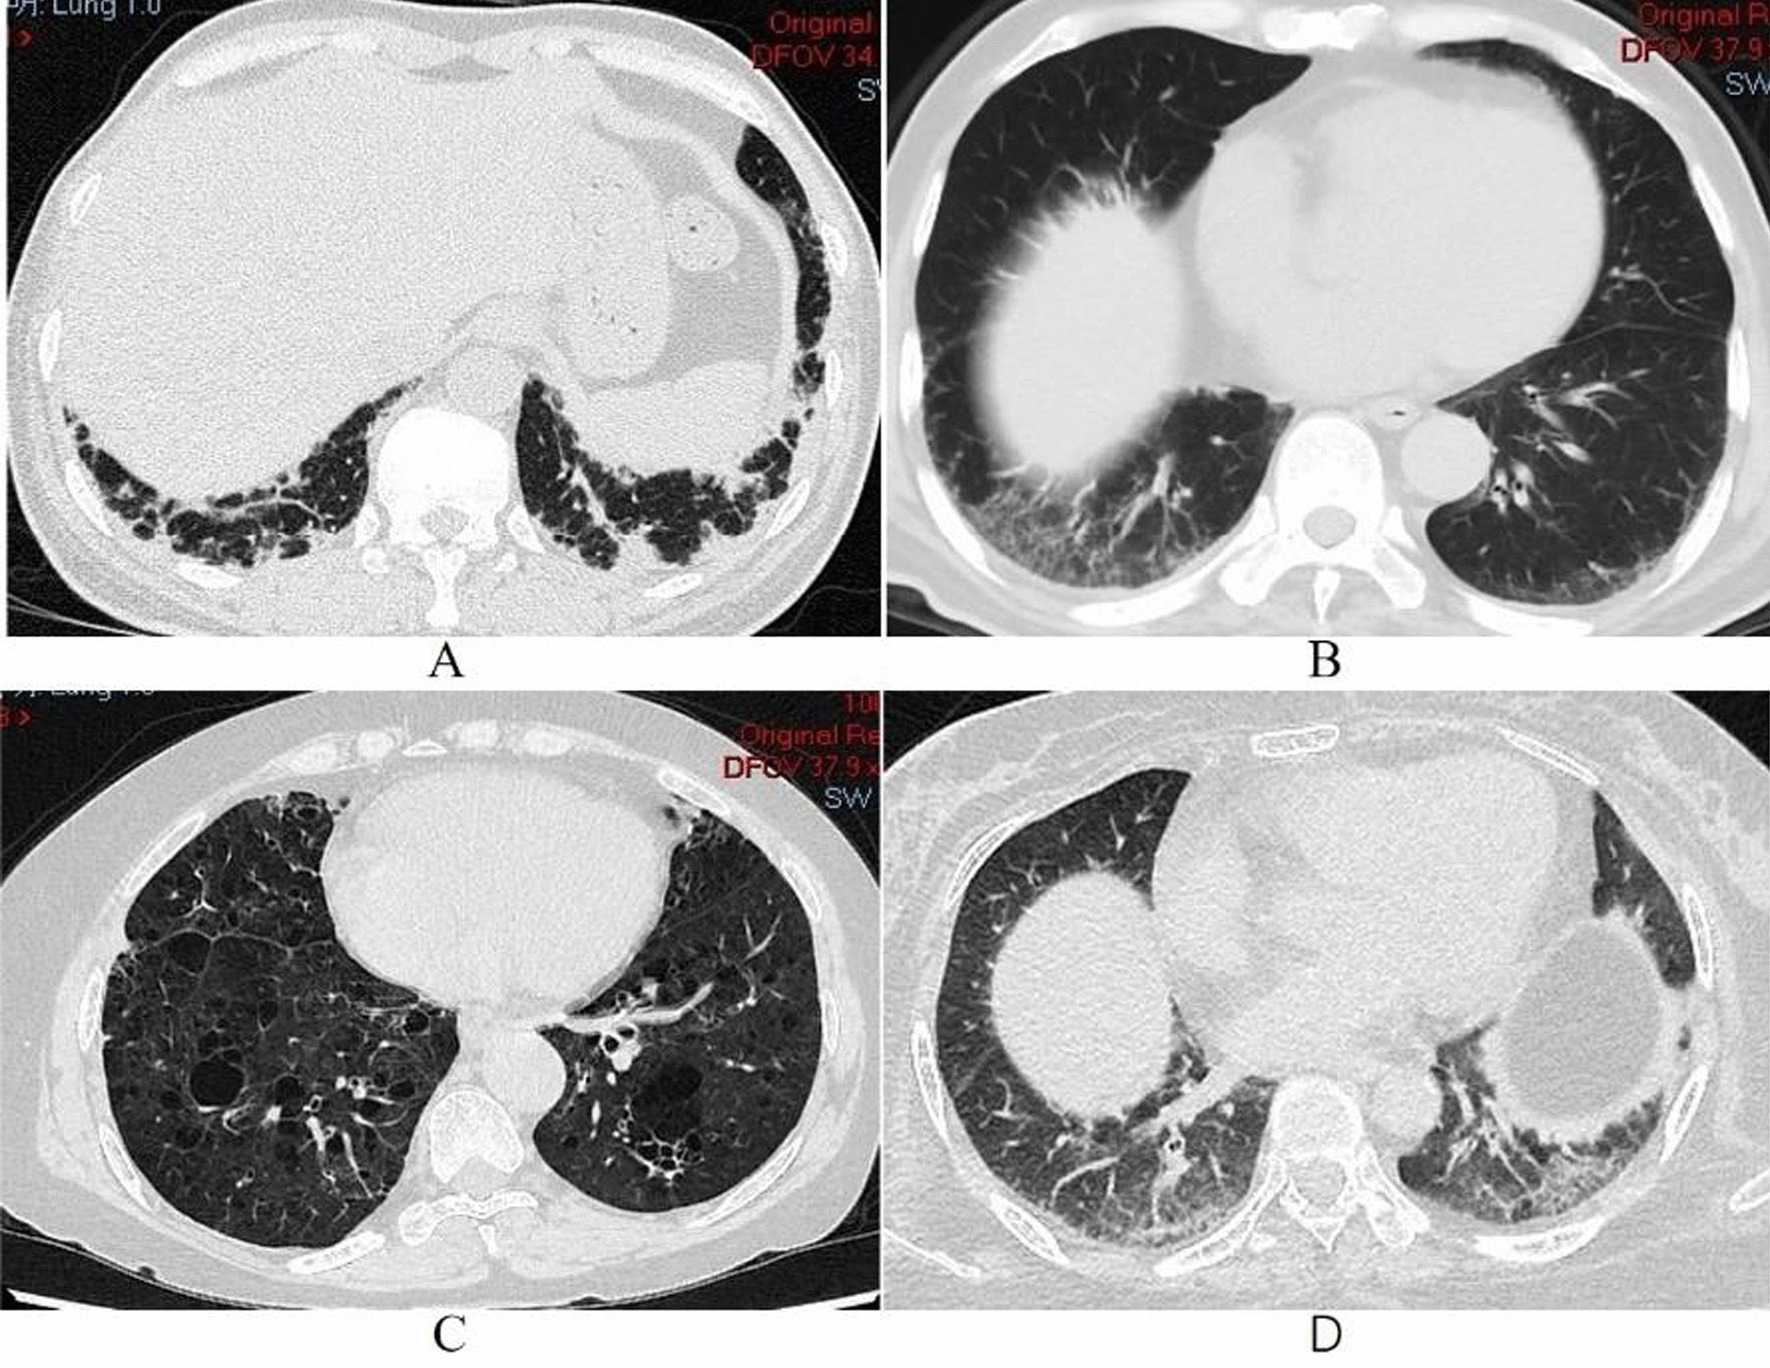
Abnormal opacities of HRCT from different SRP-IMNM patients. A 45-year-old woman with OP; B 54-year-old man with NSIP; C 55-year-old woman with LIP; D 45-year-old woman with NSIP
